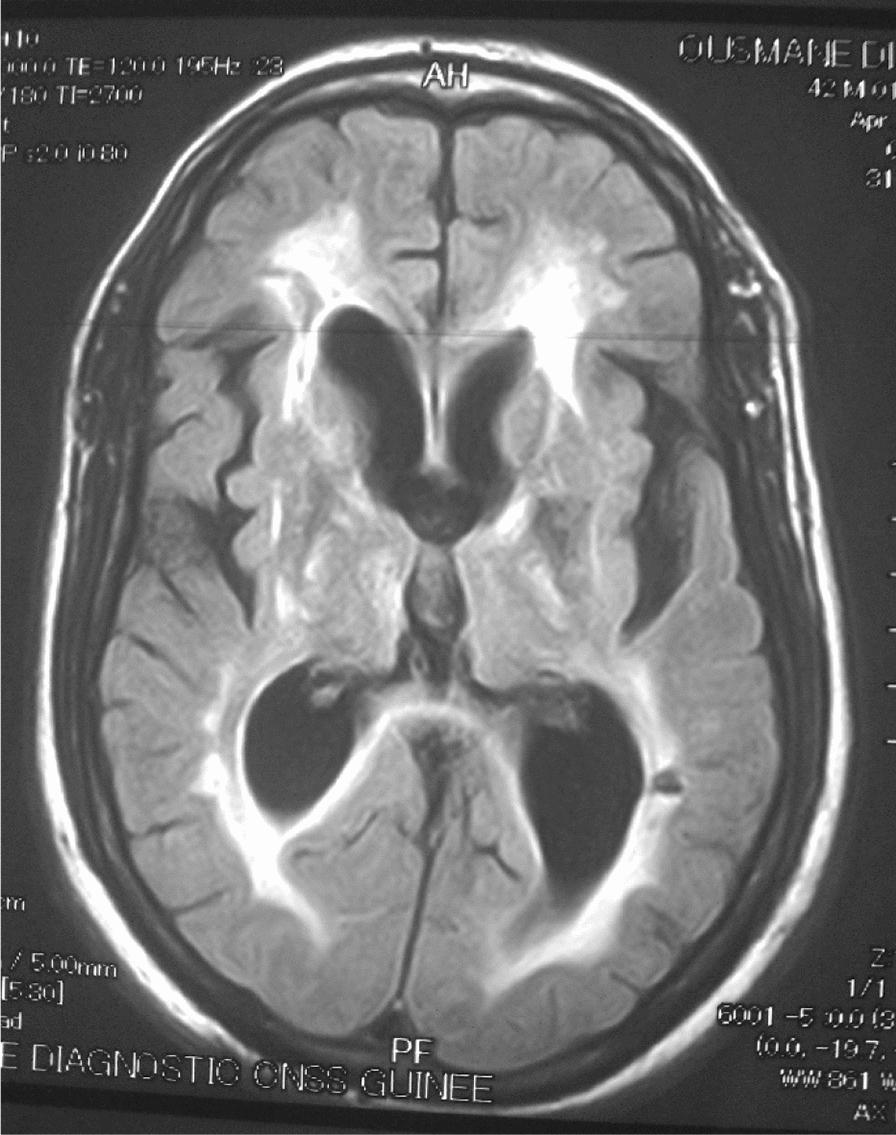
Brain MRI: axial T1 section showing areas of white matter signal abnormality
